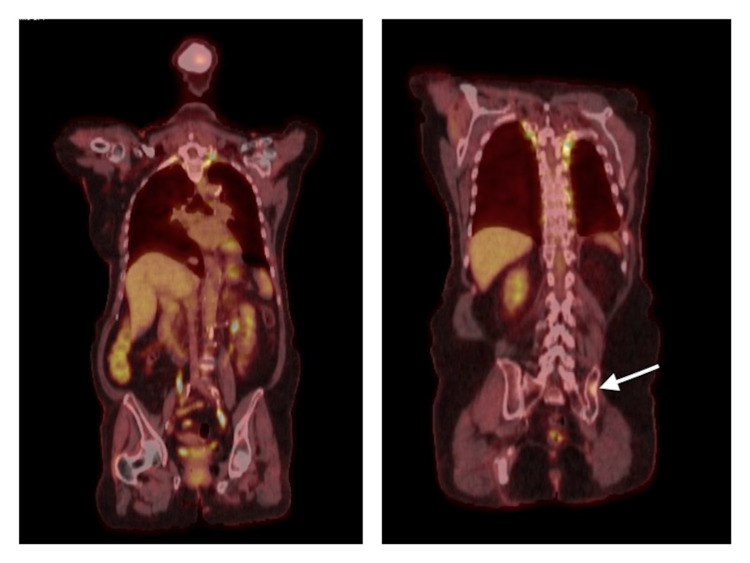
PET-CT scan from June 2020 Left: PET-CT scan from June 2020 following initiation of systemic chemotherapy, revealing resolution of left hilar and subcarinal FDG-avid lesions without new hypermetabolic disease. Right: PET-CT scan from June 2020 following initiation of systemic chemotherapy, demonstrating significantly decreased FDG activity within previous left iliac sclerotic lesion (as indicated by white arrow). PET-CT: positron emission tomography-computed tomography, FDG: fluorodeoxyglucose.
